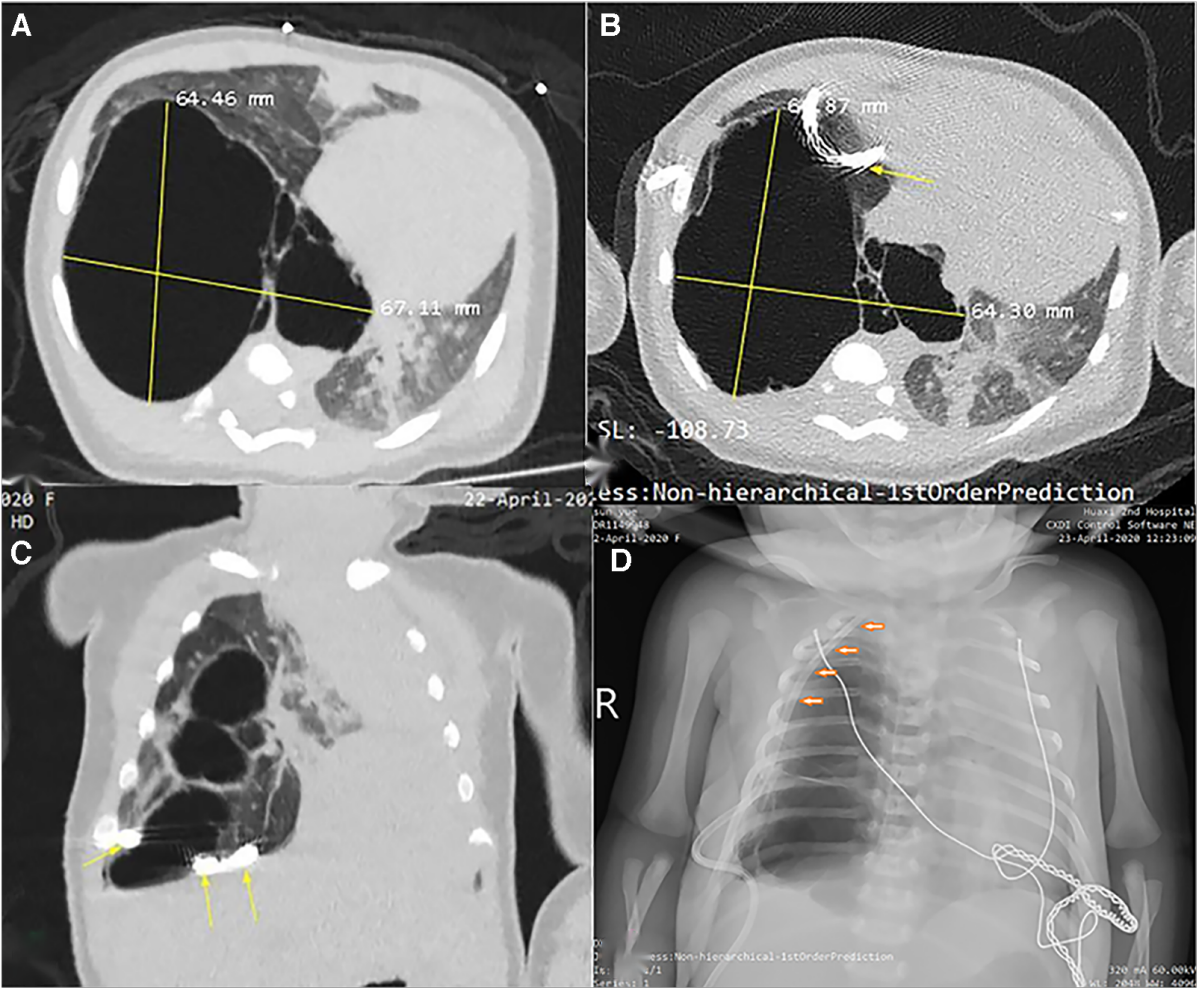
Radiological imaging of PTCD maneuvre in case 1. (A) Axial view of CT scan prior to puncture indicated a huge cystic lung lesion with septa in the right thoracic cavity; (B) and (C) CT scan demonstrated the pigtail catheter (yellow arrow) was placed (first attempt) outside of the large cyst; (D) Chest X-ray film displayed the tube was positioned into the thoracic cavity rather than the large cyst by second attempt.
